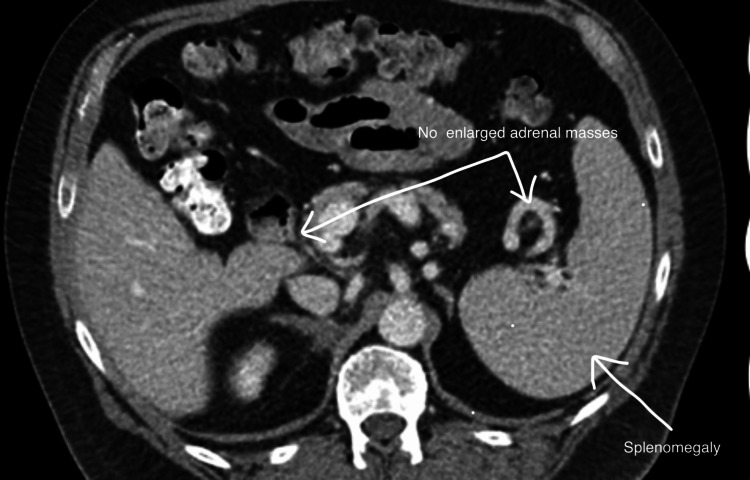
CT abdomen and pelvis post treatment No evidence of lymphoma relapse: There is no evidence of abnormal lymphadenopathy. Normal adrenals: The spleen is a little bulky but this has not changed.
